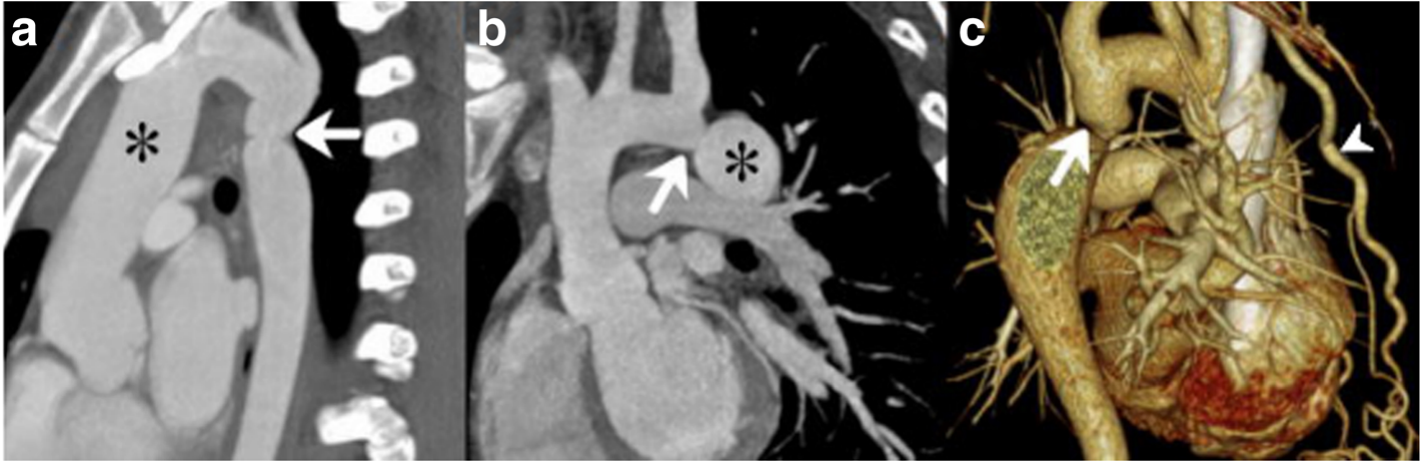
CT images of vascular abnormalities in CoA patients. Sagittal multiplanar reformatted image (a) shows a 16-year-old woman with CoA associated with dilated ascending aorta (asterisk) before the narrowing (white arrow). Sagittal multiplanar reformatted image (b) shows a 19-year-old man with CoA associated with dilated descending aorta (asterisk) after the narrowing (white arrow). Volume rendering image (c) shows a 53-year-old CoA woman with the narrowing (white arrow) and collateral circulation including enlarged internal mammary artery (arrowhead). Aortic dilation was considered if the z-score > 2
